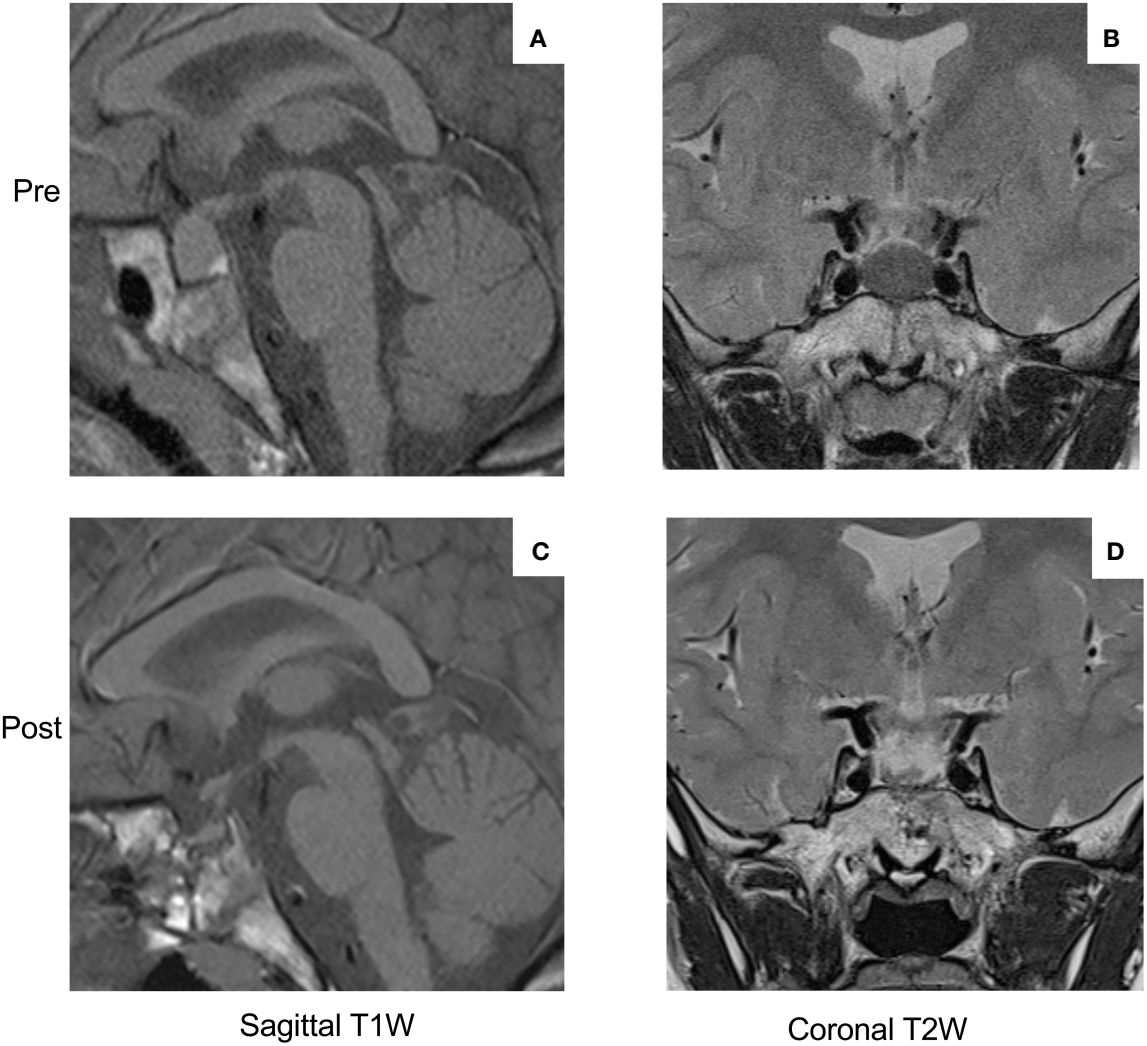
MRI images of the pituitary macroadenoma (17 × 12 mm) at diagnosis (sagittal T1-weighted image (A), coronal T2-weighted image (B)). Note the hypo-intensity of the T2-weighted image as compared with the temporal lobe gray matter. The post-operative MRI images ((C) sagittal T1-weighted; (D) T2-weighted coronal)) show the effect of gross visual resection of the pituitary adenoma and a remaining tiny region of normal anterior pituitary tissue.
